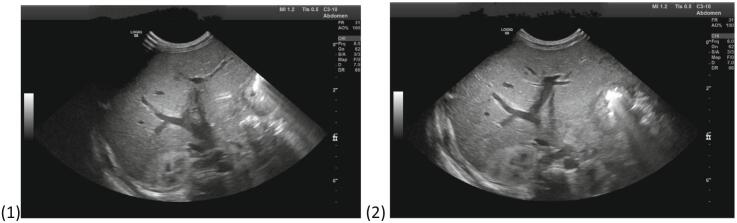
Abdominal ultrasound images 1-week post-surgery.
